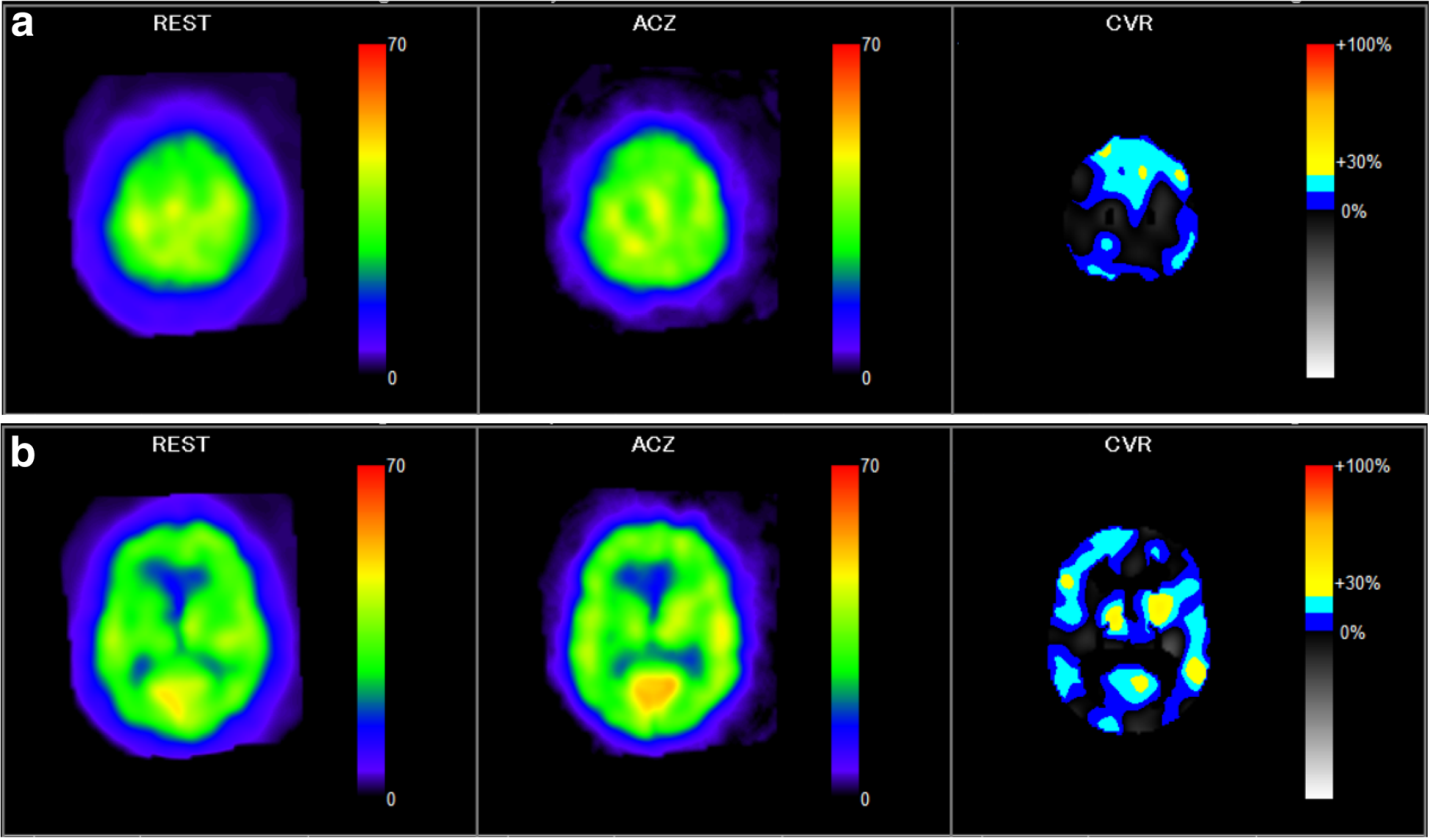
Single-photon emission computed tomography (SPECT). Quantitative CBF assessment with SPECT showed a mild decrease in resting CBF and a severe decrease in CVR over almost all the cerebral area in an acetazolamide-tolerance test. Images are shown at the level of (a) the centrum semiovale and (b) the basal ganglia. ACZ; acetazolamide, CVR; cerebrovascular reactivity
